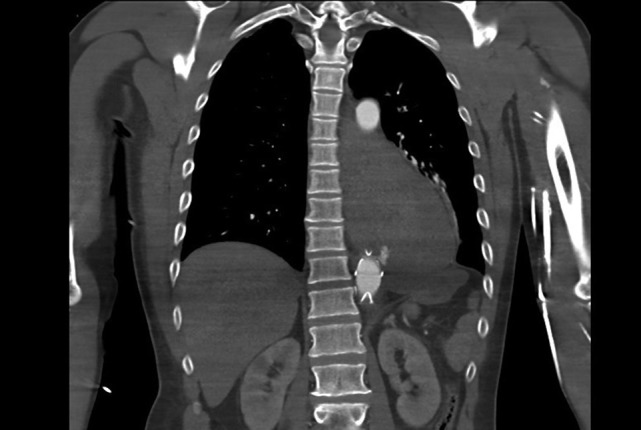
CT aortogram showing the extravasation of contrast from the aorta just proximal to the stent graft.
